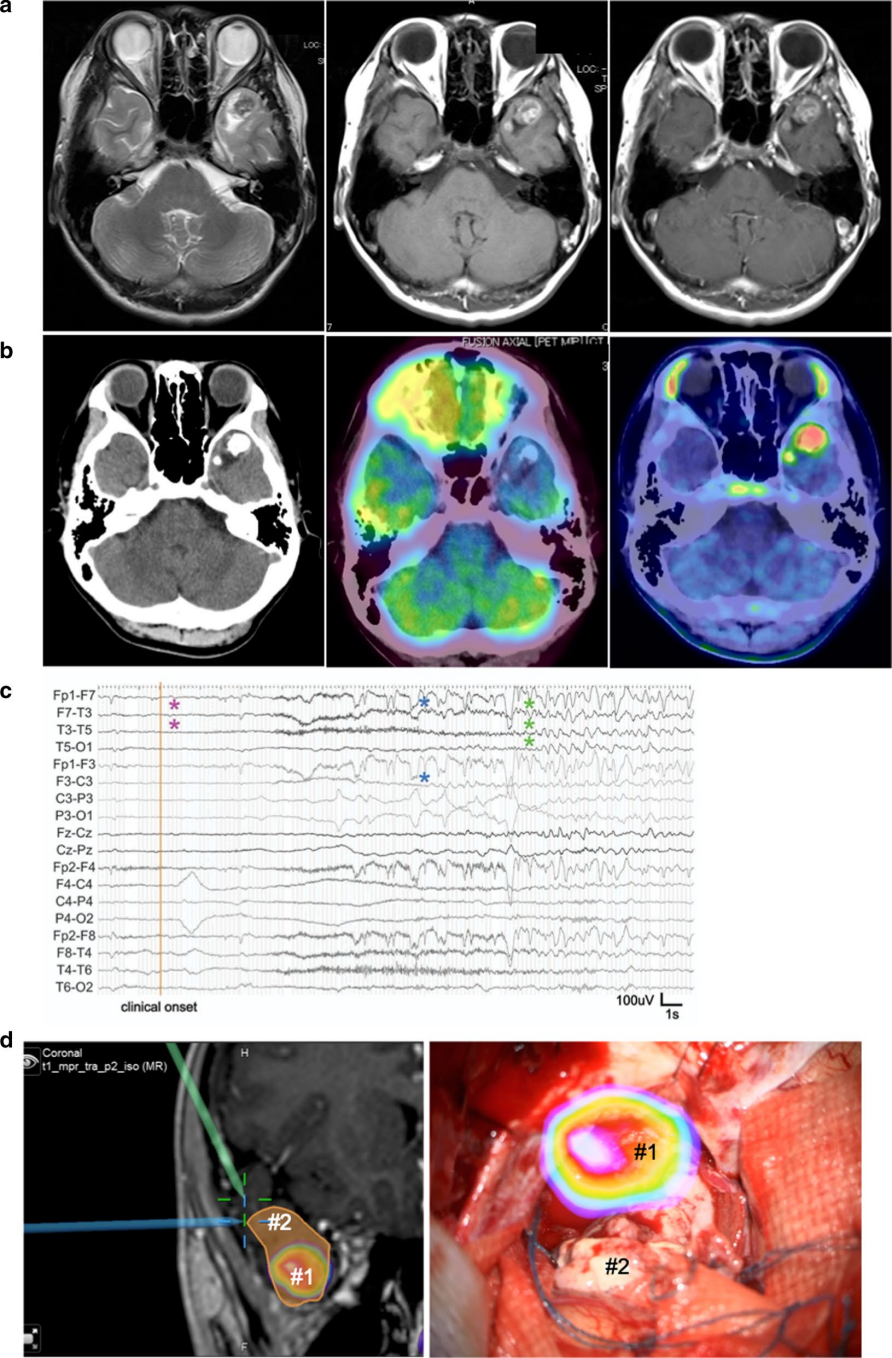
Characteristics of a patient with PLNTY. a T2-weighted (left), T1-weighted (middle), and contrast-enhanced (right) MR images. b Computed tomography (CT, left), ^18^F-fluorodeoxyglucose-PET/CT (middle), and ^11^C-methionine-PET/CT (right) images. c Video electroencephalography indicating ictal onset in the left temporal lobe, with spread to the contralateral temporal lobe. d PET/CT and MRI merged intraoperative navigation image (left) and surgical image (right) showing the high-methionine-uptake region (#1) and surrounding abnormal lesion (#2) on MRI
